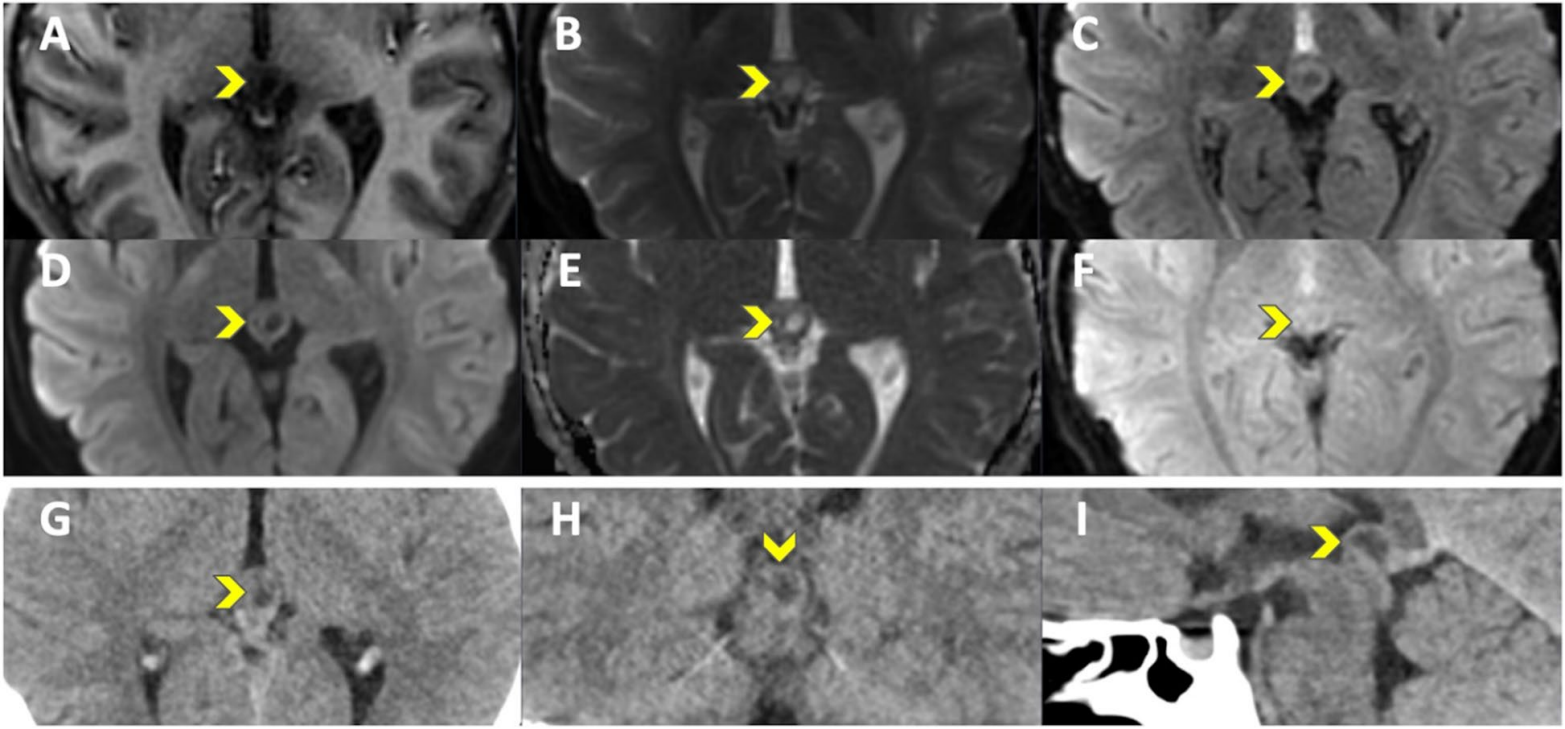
ID2. A pineal cyst (yellow head arrow), visible on both EPIMix and CT at the side-by-side consensus. T1-FLAIR (A), T2 (B), T2-FLAIR (C), DWI (D), ADC (E), T2* (F) EPIMIX images, and axial (G), coronal (H), sagittal (I) soft tissue window CT images
